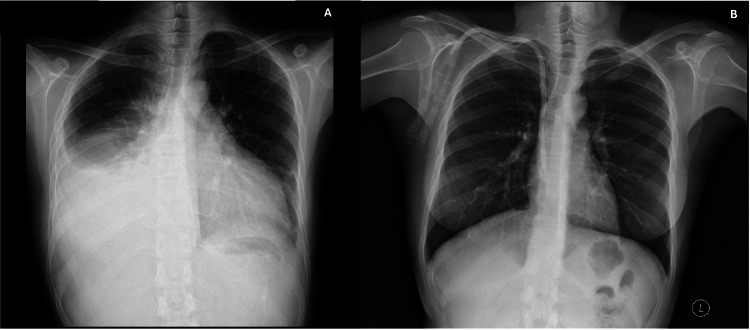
Chest X-ray before and after therapy (A) Posteroanterior chest X-ray before the start of therapy showing recurrent right pleural effusion and increased cardiothoracic index. (B) Posteroanterior chest X-ray six months after initiation of ARNi therapy, demonstrating resolution of pleural effusion and placement of a right jugular vein dialysis catheter as an alternative access following closure of the high-output fistula. ARNi, angiotensin receptor-neprilysin inhibitor
